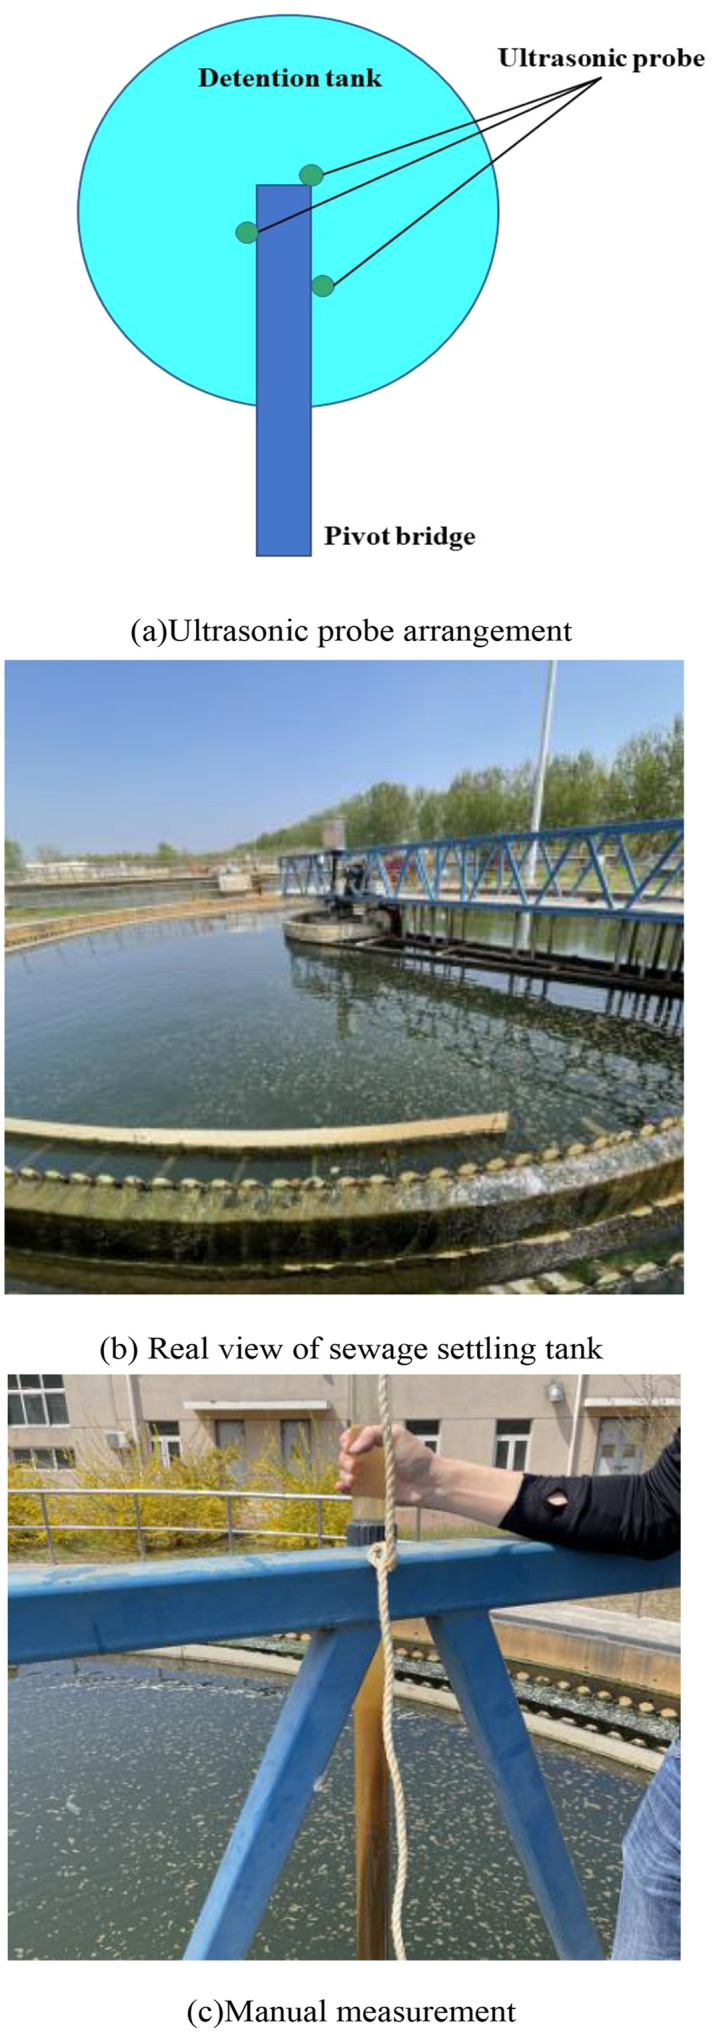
Experimental set up. (a) Ultrasonic probe arrangement. (b) Real view of sewage settling tank. (c) Manual measurement.
